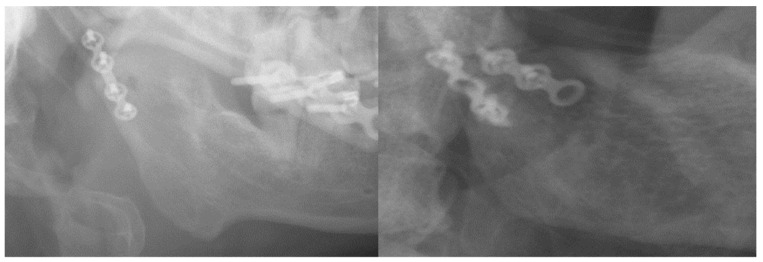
Demonstration of single-long-plate and double-short-plate fixations on a panoramic X-ray.
